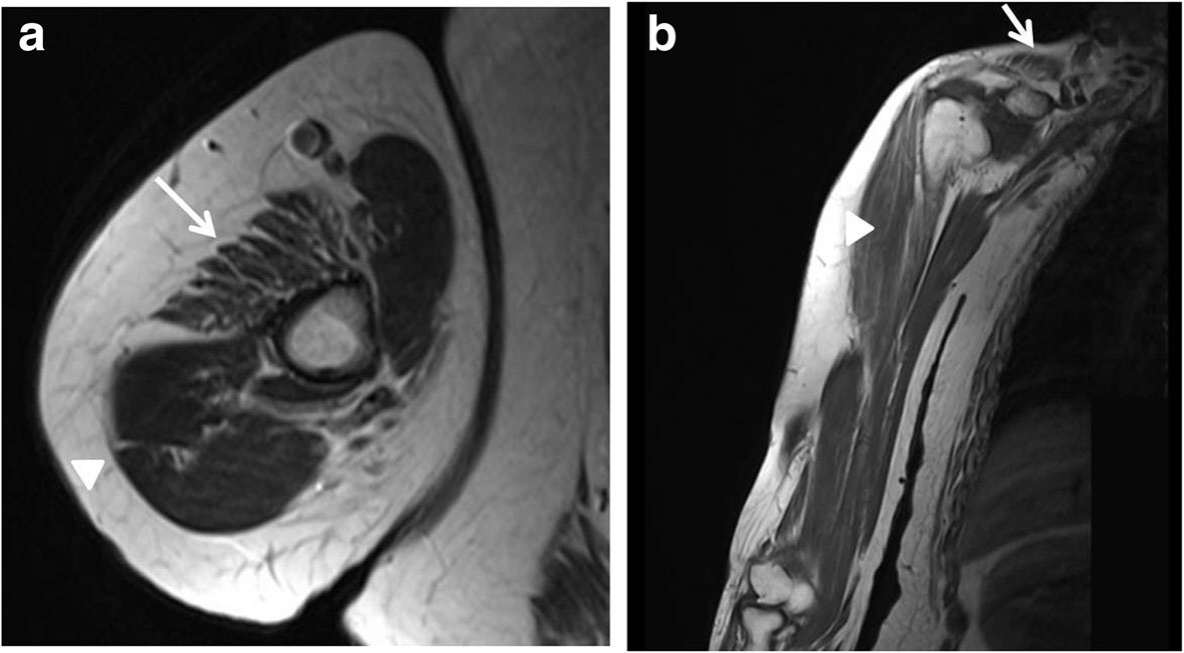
MRI of right upper extremity of the index patient. a Coronal T1 weighted MRI shows atrophy and fatty infiltration of the deltoid (arrow), and biceps brachii (arrowhead) muscles. b Sagittal T1 weighted MRI displays concomitant atrophy and fatty infiltration of the trapezius (arrows) and biceps brachii (arrowhead) muscles
